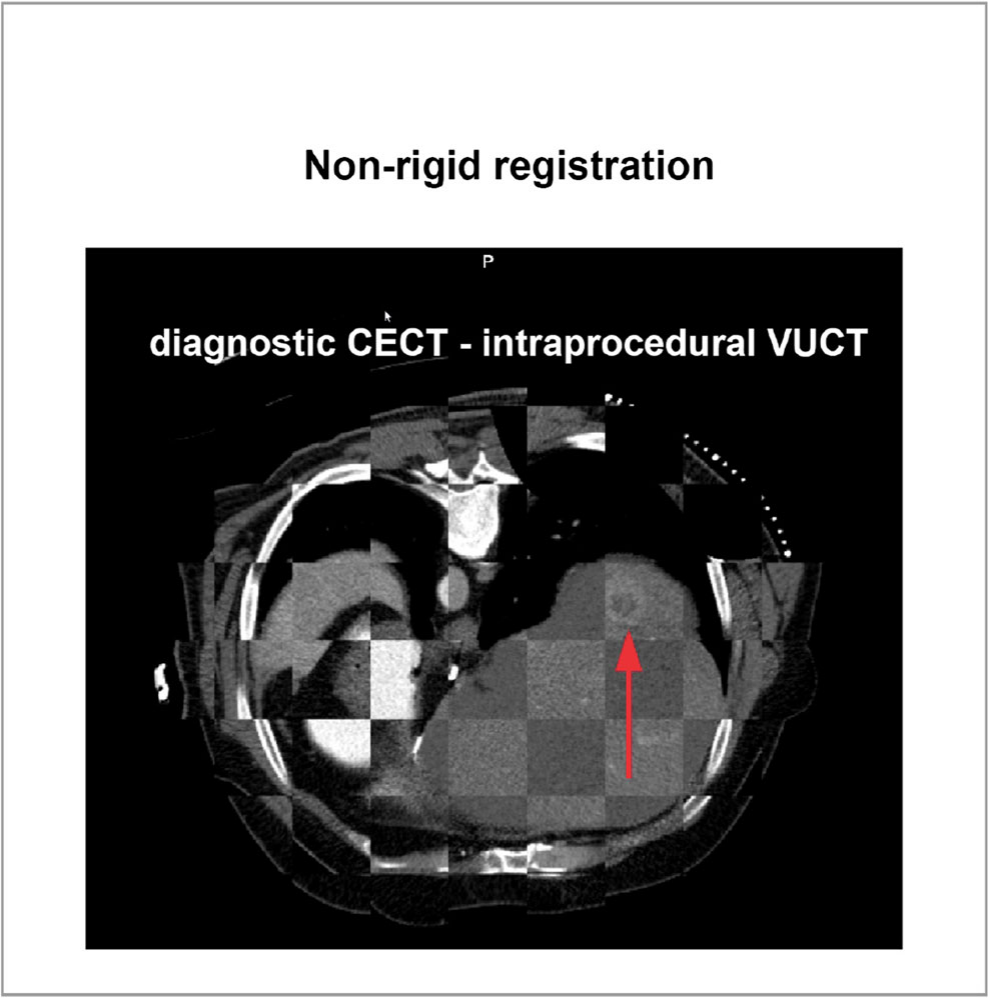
Non-rigid registration of a diagnostic CECT with its corresponding VUCT. The target tumour from the diagnostic CECT was anatomically matched into the intraprocedural VUCT (*red arrow*). Note that spatial alignment was restricted to the liver regions. *CECT* contrast-enhanced CT image, *VUCT* virtual unenhanced CT image
